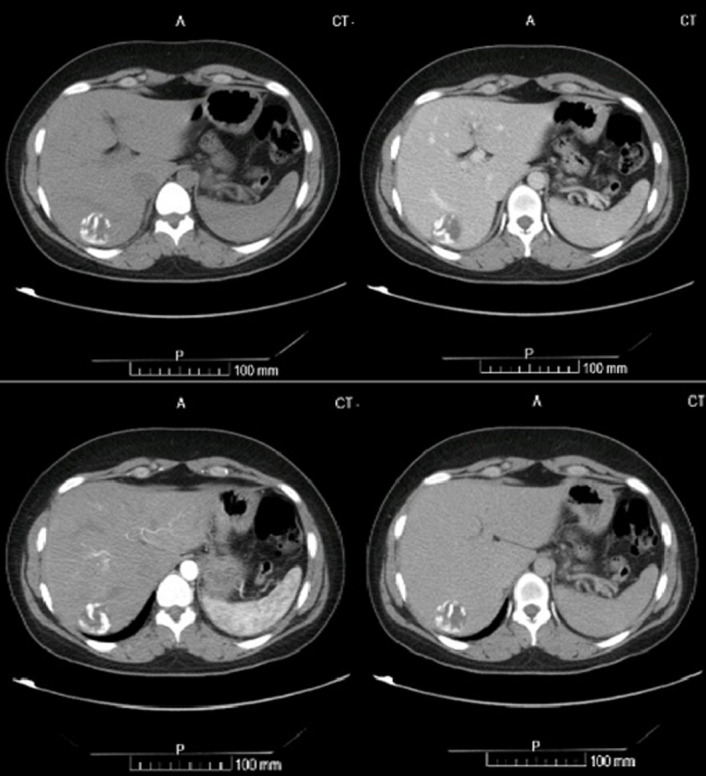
CT abdomen (axial section) showing a calcified hydatid cyst in the liver from left to right: pre-contrast, venous, arterial, delayed phase of the liver mass
